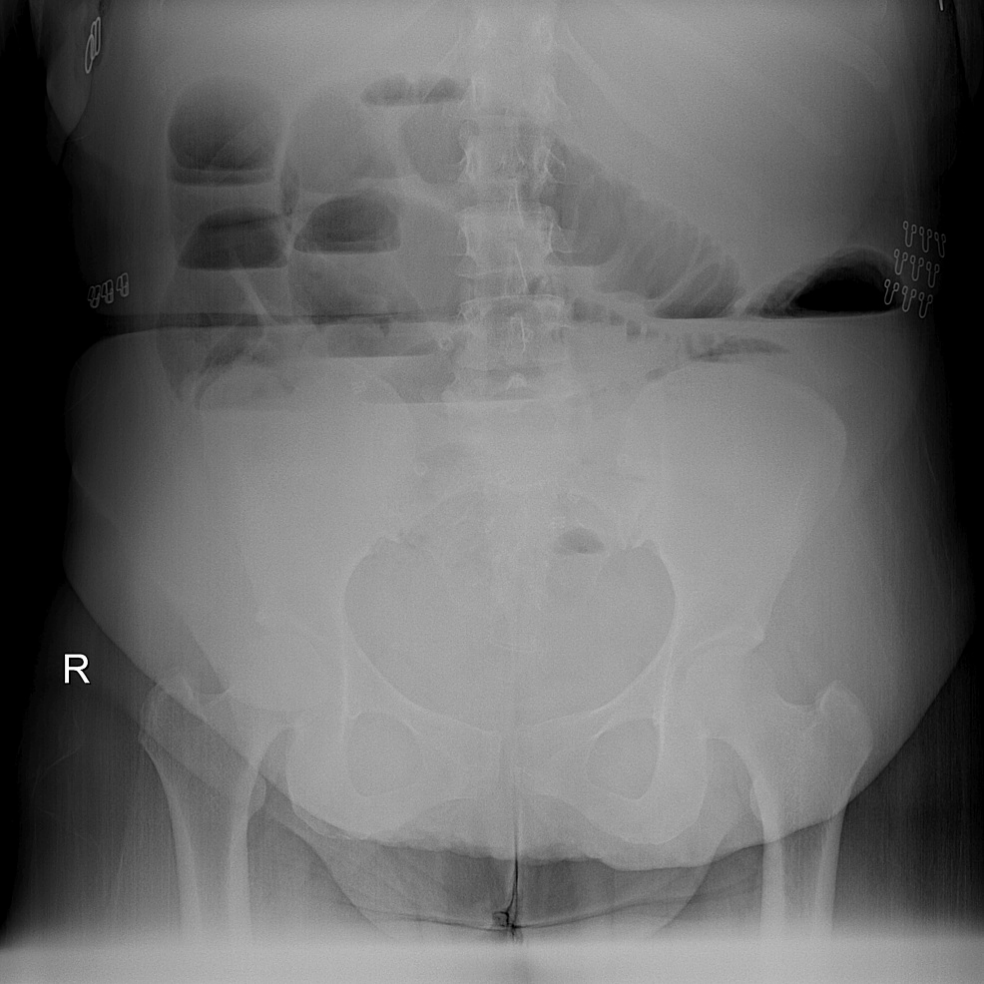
Erect abdominal X-ray shows air-fluid levels
